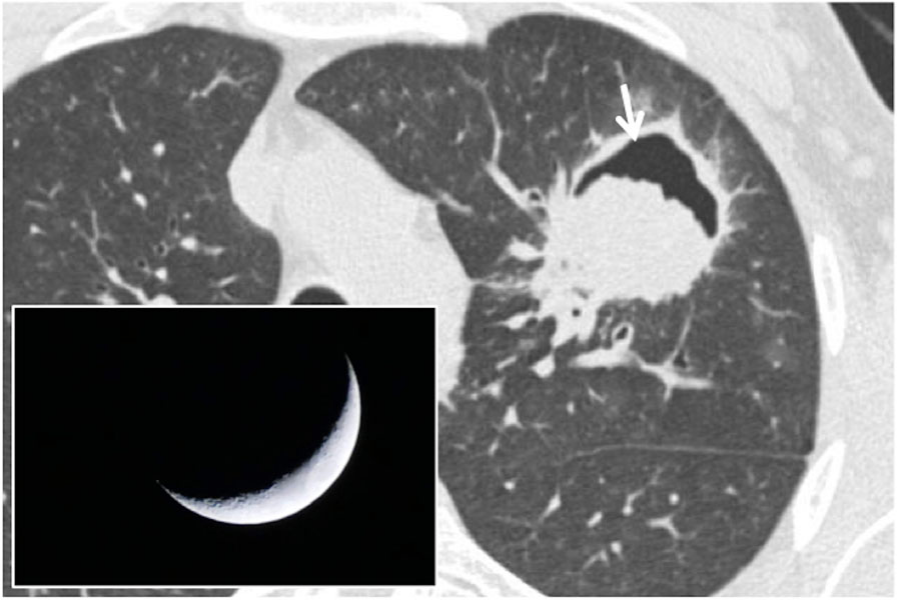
Air crescent sign. CT scan of a patient with pulmonary aspergillosis showing a necrotic and cavitated lesion (white arrow) in left upper lobe. The air filling the cavitation looks like the shadow of a crescent moon—which is demonstrated in the embedded figure
